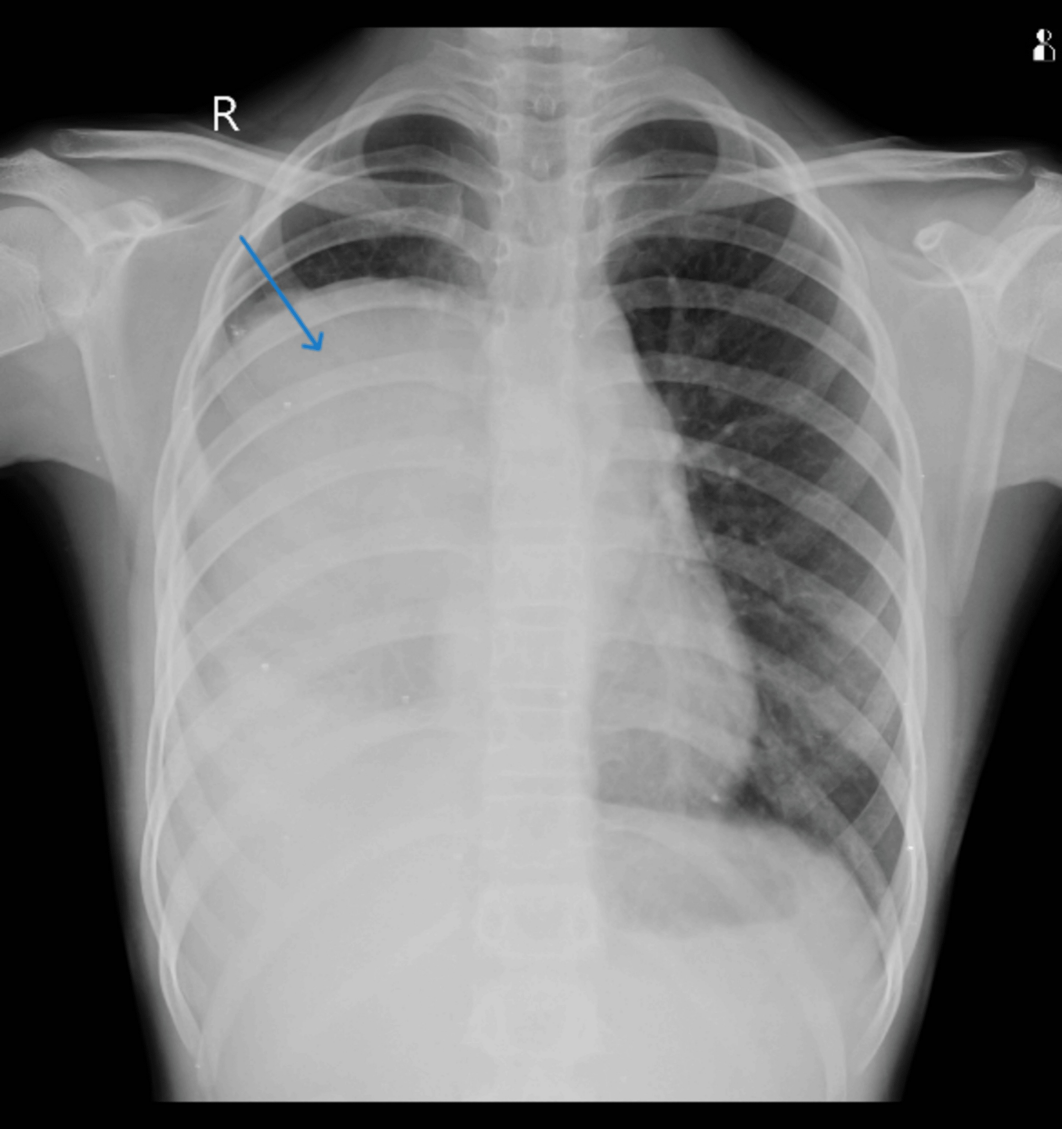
Chest radiograph PA view showing a large homogeneous opacity (blue arrow) PA, posteroanterior view.
